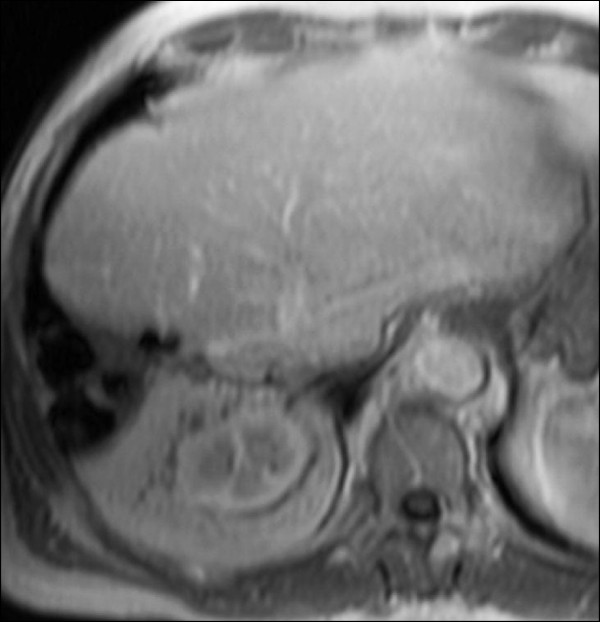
Magnetic resonance imaging of the liver transplant after resection with no evidence of recurrent disease.
